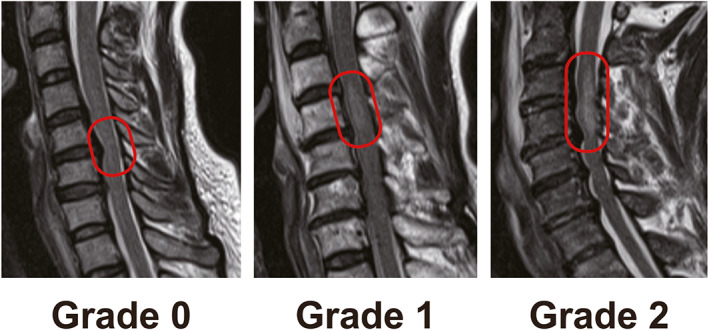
Classification of ISI of the spinal cord in T2‐weighted magnetic resonance images. ISI was classified into three grades: Grade 0, none; Grade 1, light (obscure); and Grade 2, intense (bright). ISI, increased signal intensity
